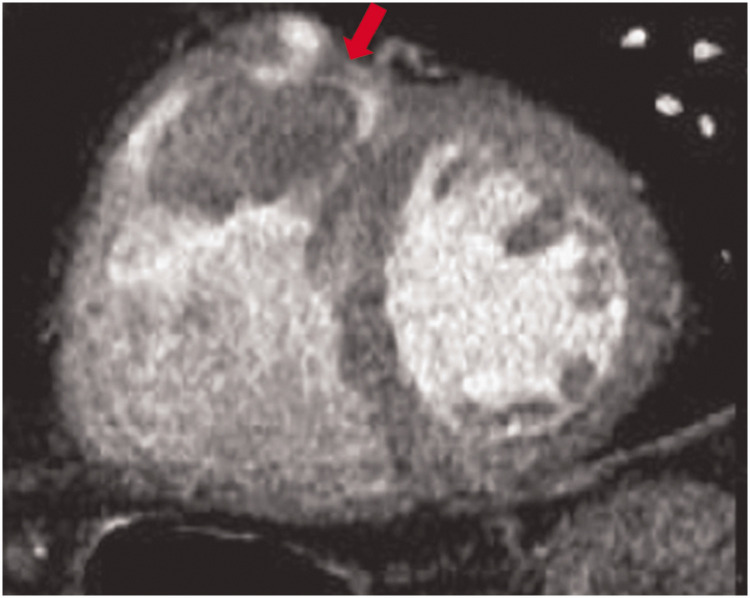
Cardiac computed tomography short-axis image shows a lobulated mass (red arrow) attached to the right ventricular outflow-tract in a patient with known hepatocellular carcinoma (used with permission).
